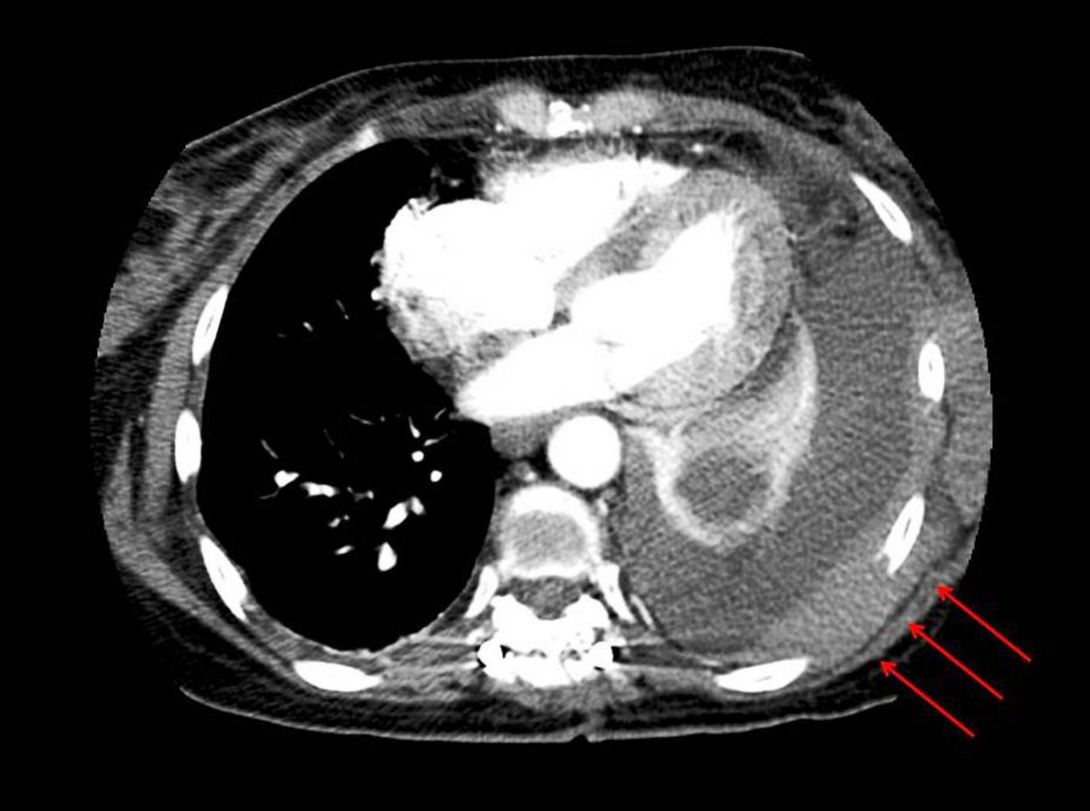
CT chest showing large pleural effusion with collapsed lobe of the lung, right mediastinal shift, and pleural thickening due to metastasis to pleura (marked by red arrows).
